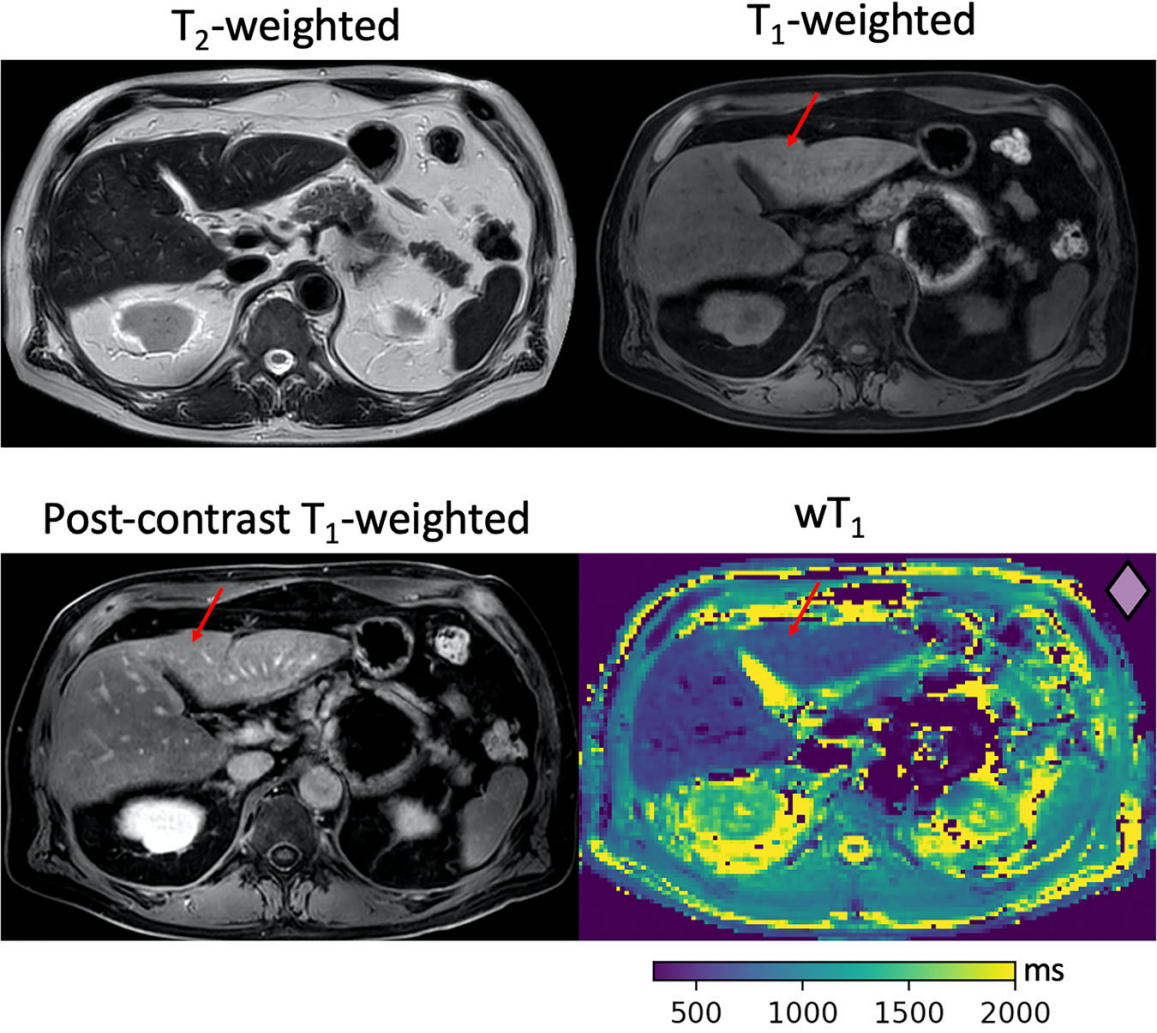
A 62-year-old male with idiopathic granulomatous hepatitis. The T2-weighted image shows no abnormalities. Slight and mild T1 hyperintensity in S4a/b (arrow) is shown on the pre- and post-contrast T1-weighted image, respectively. Remarkably, the wT1 map reveals subtle, diffuse signal enhancement in S4a/b (arrow) without the use of a contrast agent. The wT1 and wT1_comp_ values of S4a/b (wT1_S4a_ = 814 ms, wT1_comp-S4a_ = 1,000 ms, wT1_S4b_ = 810 ms, wT1_comp-S4b_ = 960 ms) are represented as a thick-edged rhombus (right upper corner wT1 map) in Fig. 4b. wT1, Water T1; wT1_comp_, T2*-compensated wT1
